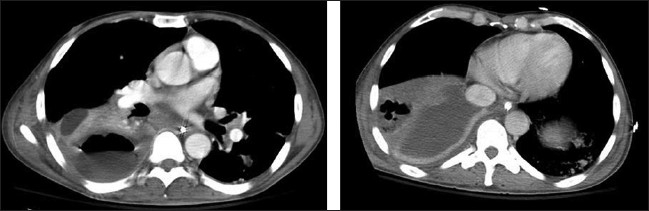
Same patient as in Figure 8; the opacity is in the peripheral lung, near the injured chest wall. The lesion rapidly progresses to cavitation as seen on the axial CT scans. The appearances are those of a lung contusion. Contusion however usually occurs earlier, is usually localized to the area affected by injury (e.g., unilateral and lower or upper zones) and improves over 48-72 hours. ARDS tends to be more generalized, is later in onset and slower to resolve
